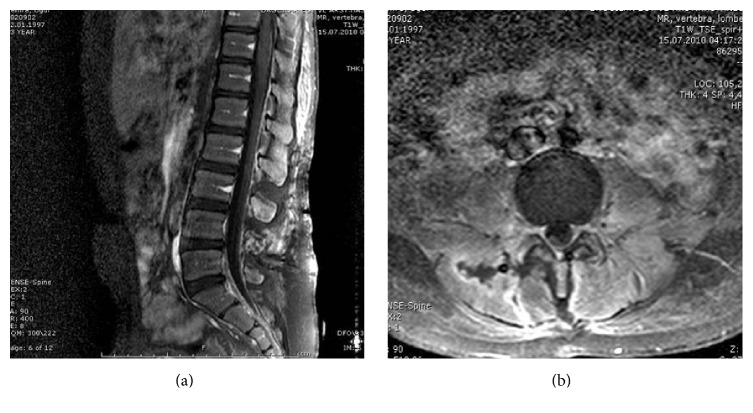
(a) Postoperative sagittal MRI. (b) Postoperative axial MRI with contrast.
